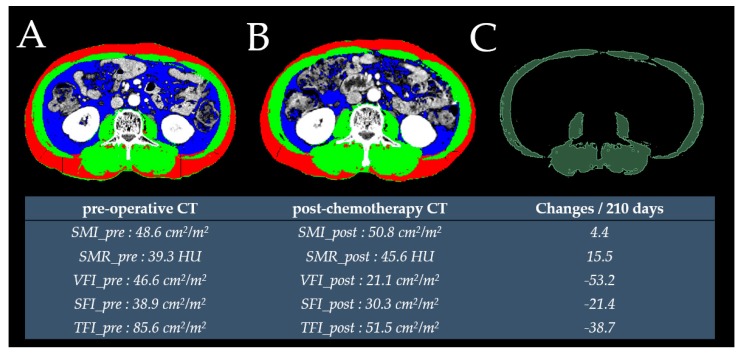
Cross-sectional L3 CT image used to measure subcutaneous fat (red), skeletal muscle (green) and visceral fat (blue) using preoperative (A) and postchemotherapy CT (B) images. The radiodensity of skeletal muscle in the preoperative CT was measured using an open-source three-dimensional slicer software (C).
